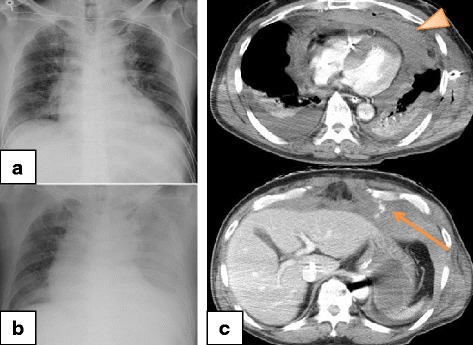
Chest radiography and computed tomography findings from Patient 2. a Chest radiography at the time of admission did not reveal any traumatic abnormalities. b Chest radiography 3 days after the admission revealed decreased left lung permeability. c Contrast-enhanced computed tomography from after the insertion of a chest drainage tube revealed extravasation of the contrast agent behind the left sixth rib (*arrow*), a hematoma at the mediastinum (*arrowhead*), and chest cavities without any obvious rib fractures
